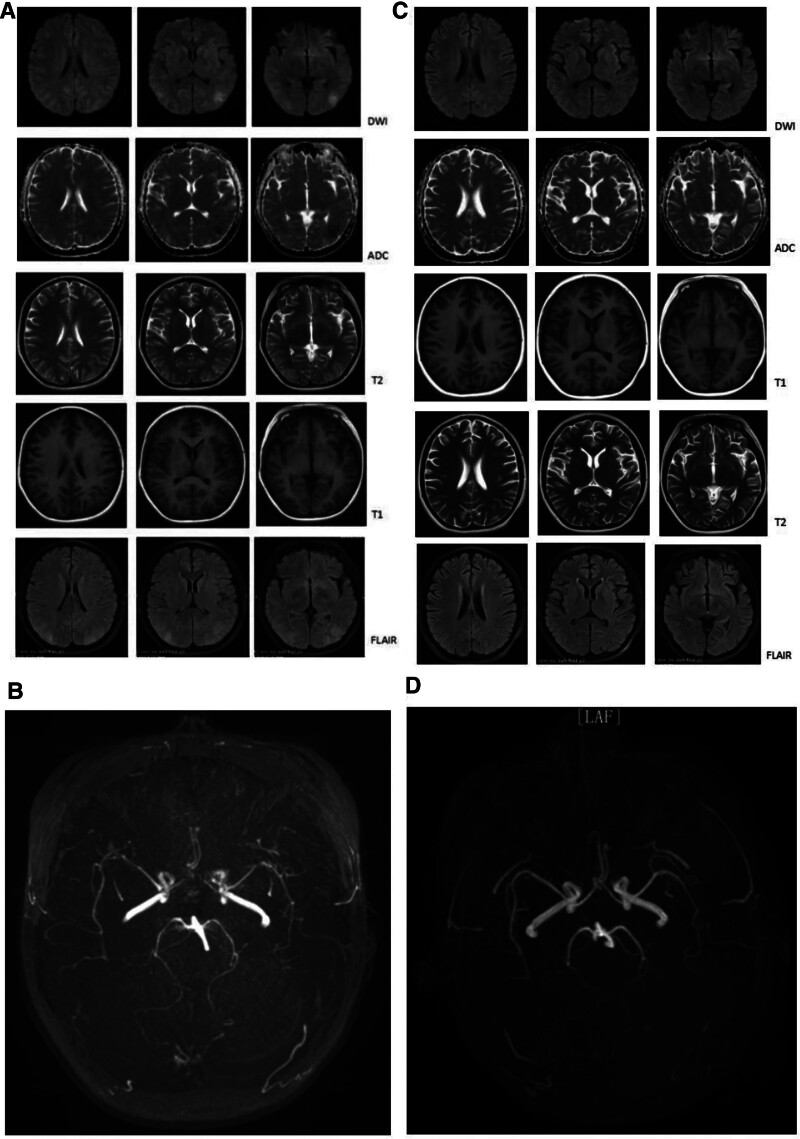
(A) Brain MRI showing hypointense signals on T1 and ADC scan in bilateral temporal, parietal, and occipital lobes while revealing hyperintense signals on T2 and FLAIR during acute attack phase. (B) MRA showing multiple segmental stenosis in bilateral anterior cerebral artery, middle cerebral artery, and posterior cerebral artery during acute attack phase. (C) Brain MRI showing disappearance of lesions in bilateral temporal, parietal, and occipital lobes during the remission period. (D) MRA revealing normal intracranial vessels during the remission period. ADC = apparent diffusion coefficient, FLAIR = fluid attenuated inversion recovery, MRA = magnetic resonance angiography, MRI = magnetic resonance imaging.
